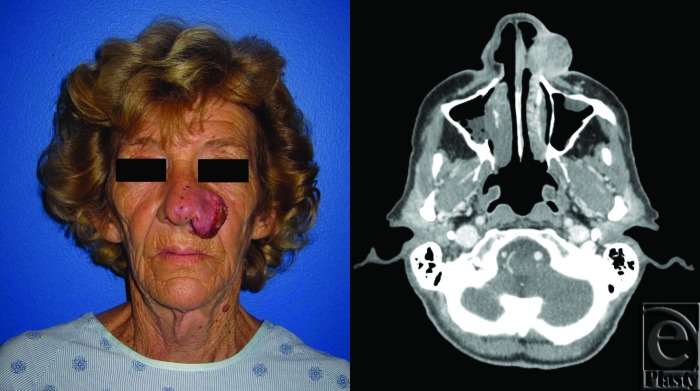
Squamous cell carcinoma of the left nose and medial cheek. Preoperative photograph (*left*). Preoperative axial computed tomographic image of invasive tumor (*right*).
